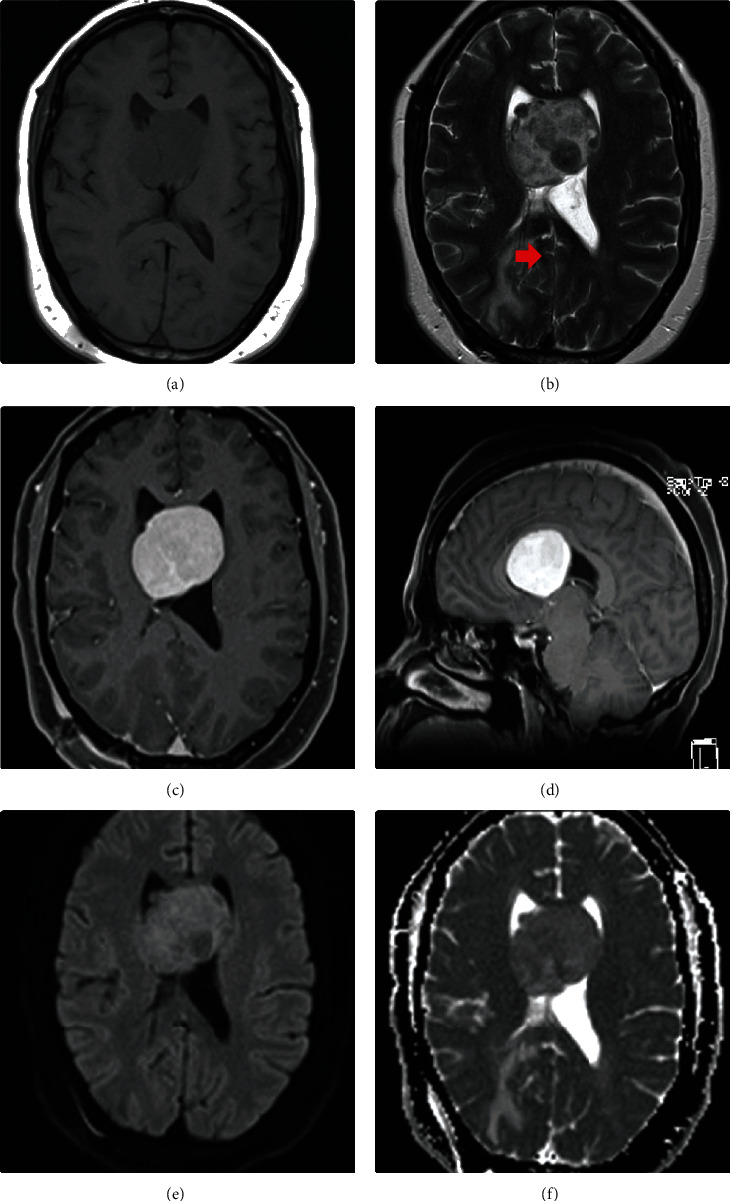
MRI images of a 57-year-old black female who presented with a history of headaches with no neurological deficits, after being involved in a pedestrian-vehicle accident. There is well well-circumscribed intraventricular lesion obstructing the foramen of Munro, with resultant hydrocephalus, thus VP shunt (red arrow) in situ. The lesion is isointense to the brain on T1 (a) and heterogeneous on T2 (b). Avid contrast enhancement is noted in (c) and (d). Diffusion-weighted imaging demonstrated restricted diffusion (e and f).
